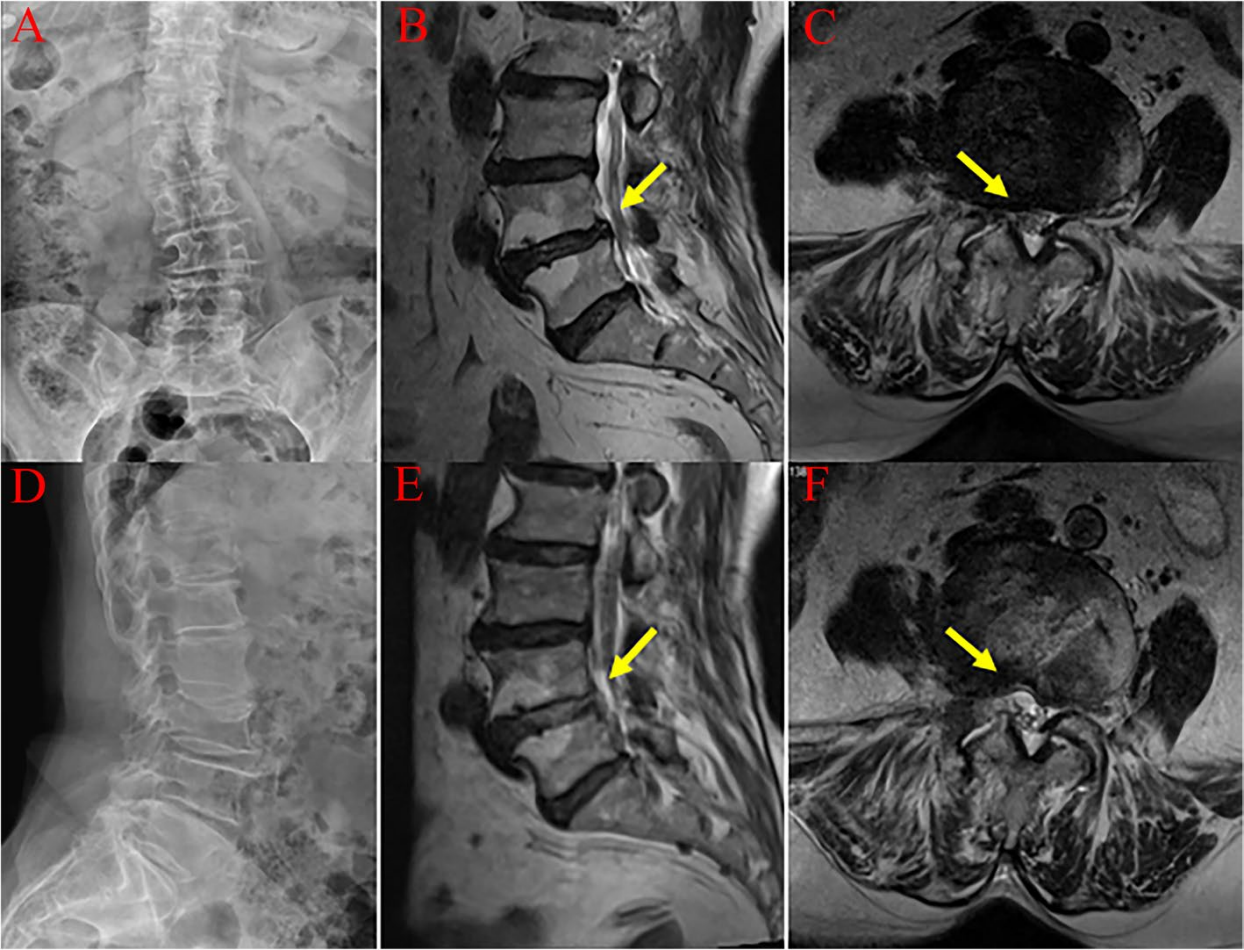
A 73-year-old male patient with a 1-year history of previous cerebral infarction. He had severe degeneration of the lumbar spine and underwent PTED surgery on the L4–5 segment. A and D are preoperative frontal and lateral radiographs, respectively. B and E are sagittal comparisons of MRI before and after surgery, respectively. E and F are axial comparisons of MRI images of the responsible segment. (Yellow arrows show the changes in the L4–5 segment before and after surgery)
